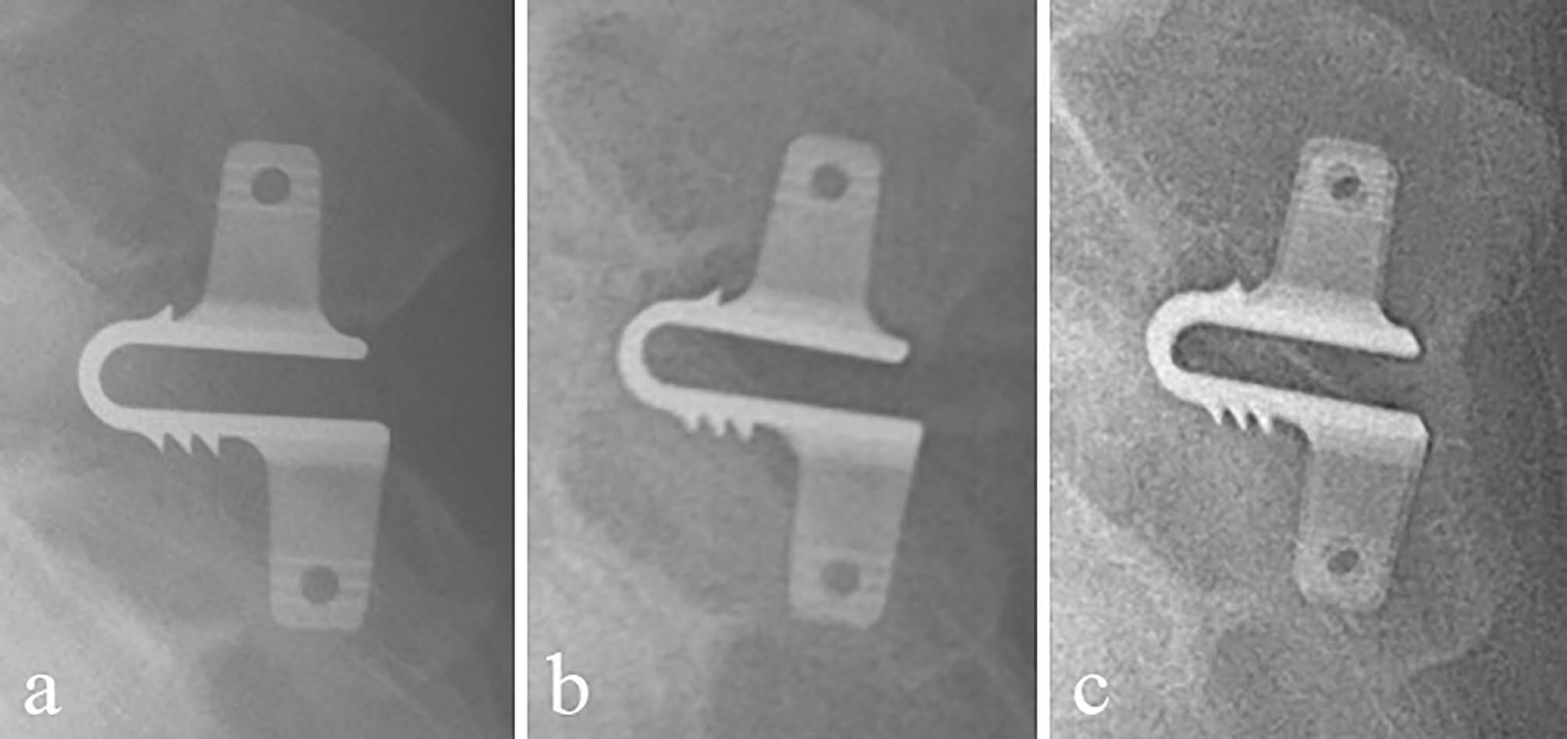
Radiographs of one patient, lateral views. a: No HO seen immediately after surgery. b: Grade 1 HO seen at one year after surgery. c: Interspinous fusion at the final follow-up visit
